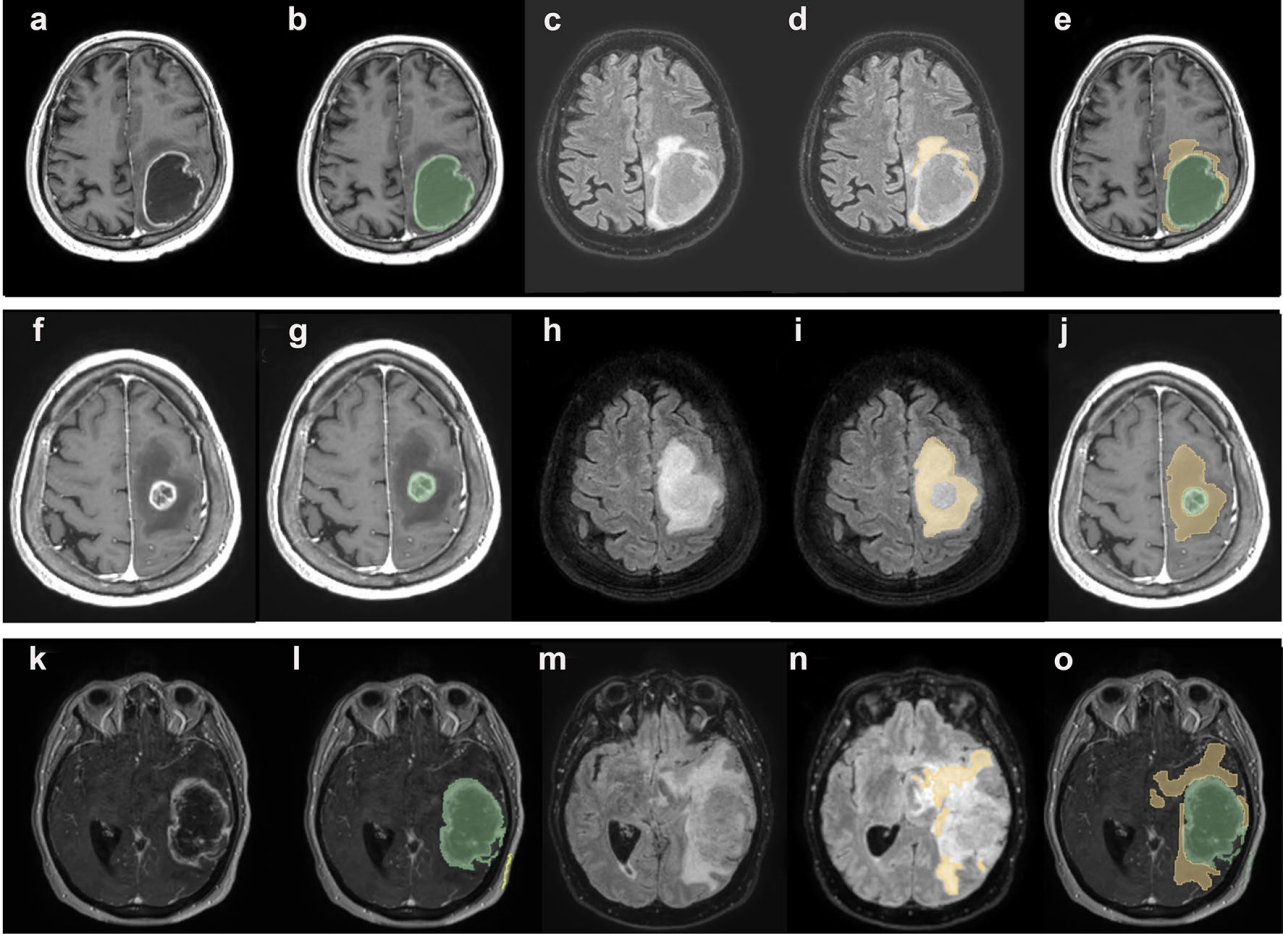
MRI of GBM biopsies for each edema index (EI) category. In the first row a glioma with EI approximately = 1; in the second row a glioma with EI > 2; in the third row a glioma with 1 < EI < 2. In a, f, k T1 with gadolinium sequence. In b, g and l tumor volume was segmented in green. In c, h and m FLAIR sequences, co-registered with T1 with gadolinium. In d, i and n edema volume was segmented in yellow. In e, j and o tumor and edema volume segmentation is represented on a T1 with gadolinium acquisition
